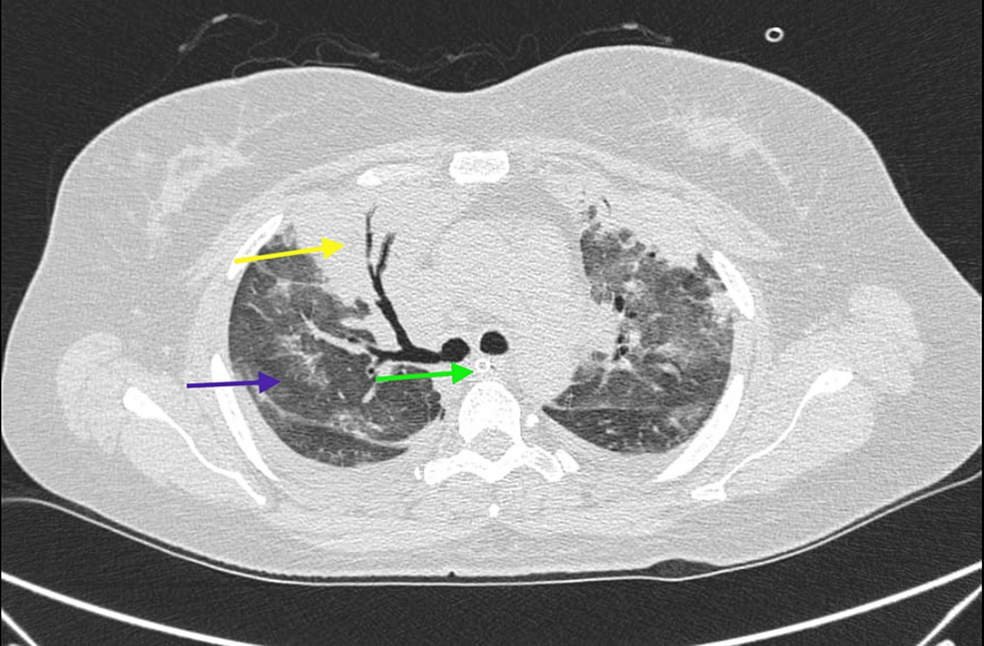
Computed tomography (CT) scan of thorax (coronal view) showing right upper lobe consolidation with air bronchogram (yellow arrow), extensive ground glass opacities with fissural effusion (purple arrow) and tracheostomy tube in situ (green arrow).
